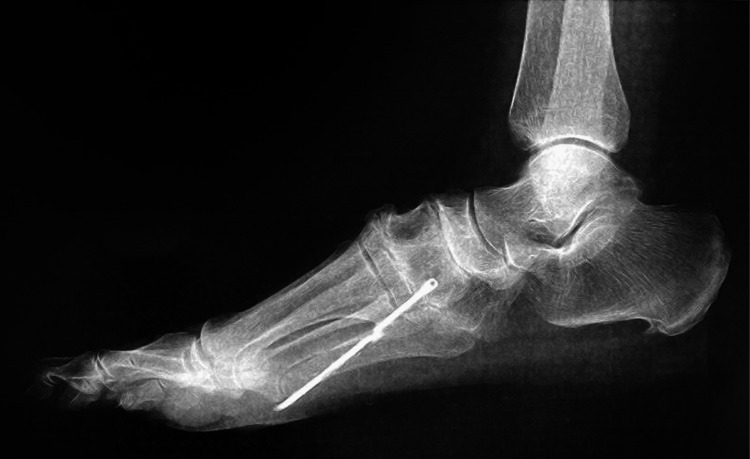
Diagnostic radiograph (lateral incidence). Foreign body projected at the base of the second metatarsal with linear metallic density, associated with small calcifications of a residual nature; plantar spur of the calcaneus; osteoarthritis of the middle foot and metatarsophalangeal and interphalangeal joint of the first toe.
